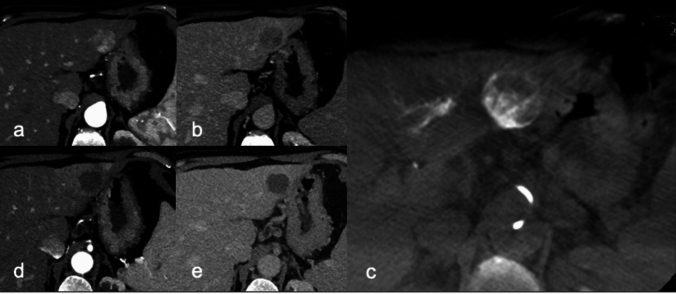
67-year-old male. Pre-procedural axial arterial (a) and delayed (b) phase of contrast-enhanced CT scan showing a 3.7 cm HCC between II-III hepatic segment. The patient underwent combined treatment: (c) the axial unenhanced CBCT image shows a complete Hug Sign—beads/trapped contrast agent completely surrounding the post-ablation volume. 3-months follow-up axial arterial (d) and delayed (e) phase contrast-enhanced CT images showing a complete response, without residual tumor. HCC: hepatocellular carcinoma. CBCT: cone-beam CT
